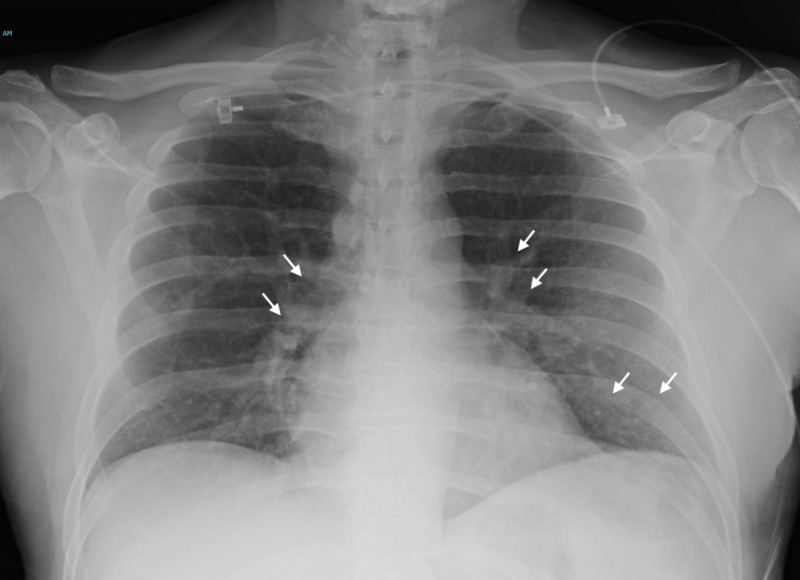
Chest x-ray showing left lower lobe infiltrates in addition to patchy left perihilar ground-glass airspace disease, and right middle lobe ground-glass airspace disease.
